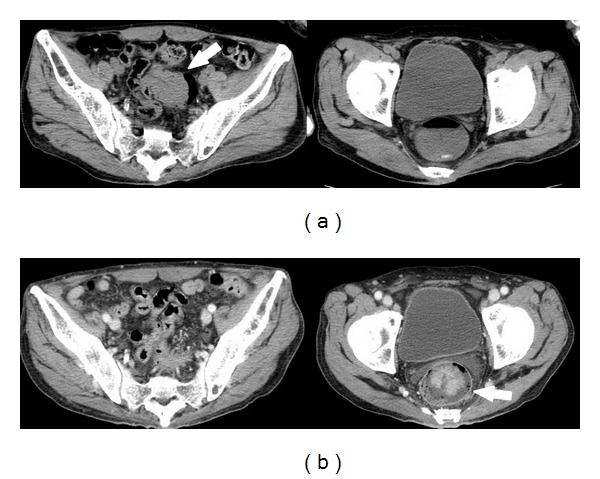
Initial CT showed the tumor located in the rectosigmoid colon (a). Preoperative contrast-enhanced CT showed that the tumor shifted to rectum below the peritoneal reflection (b).
